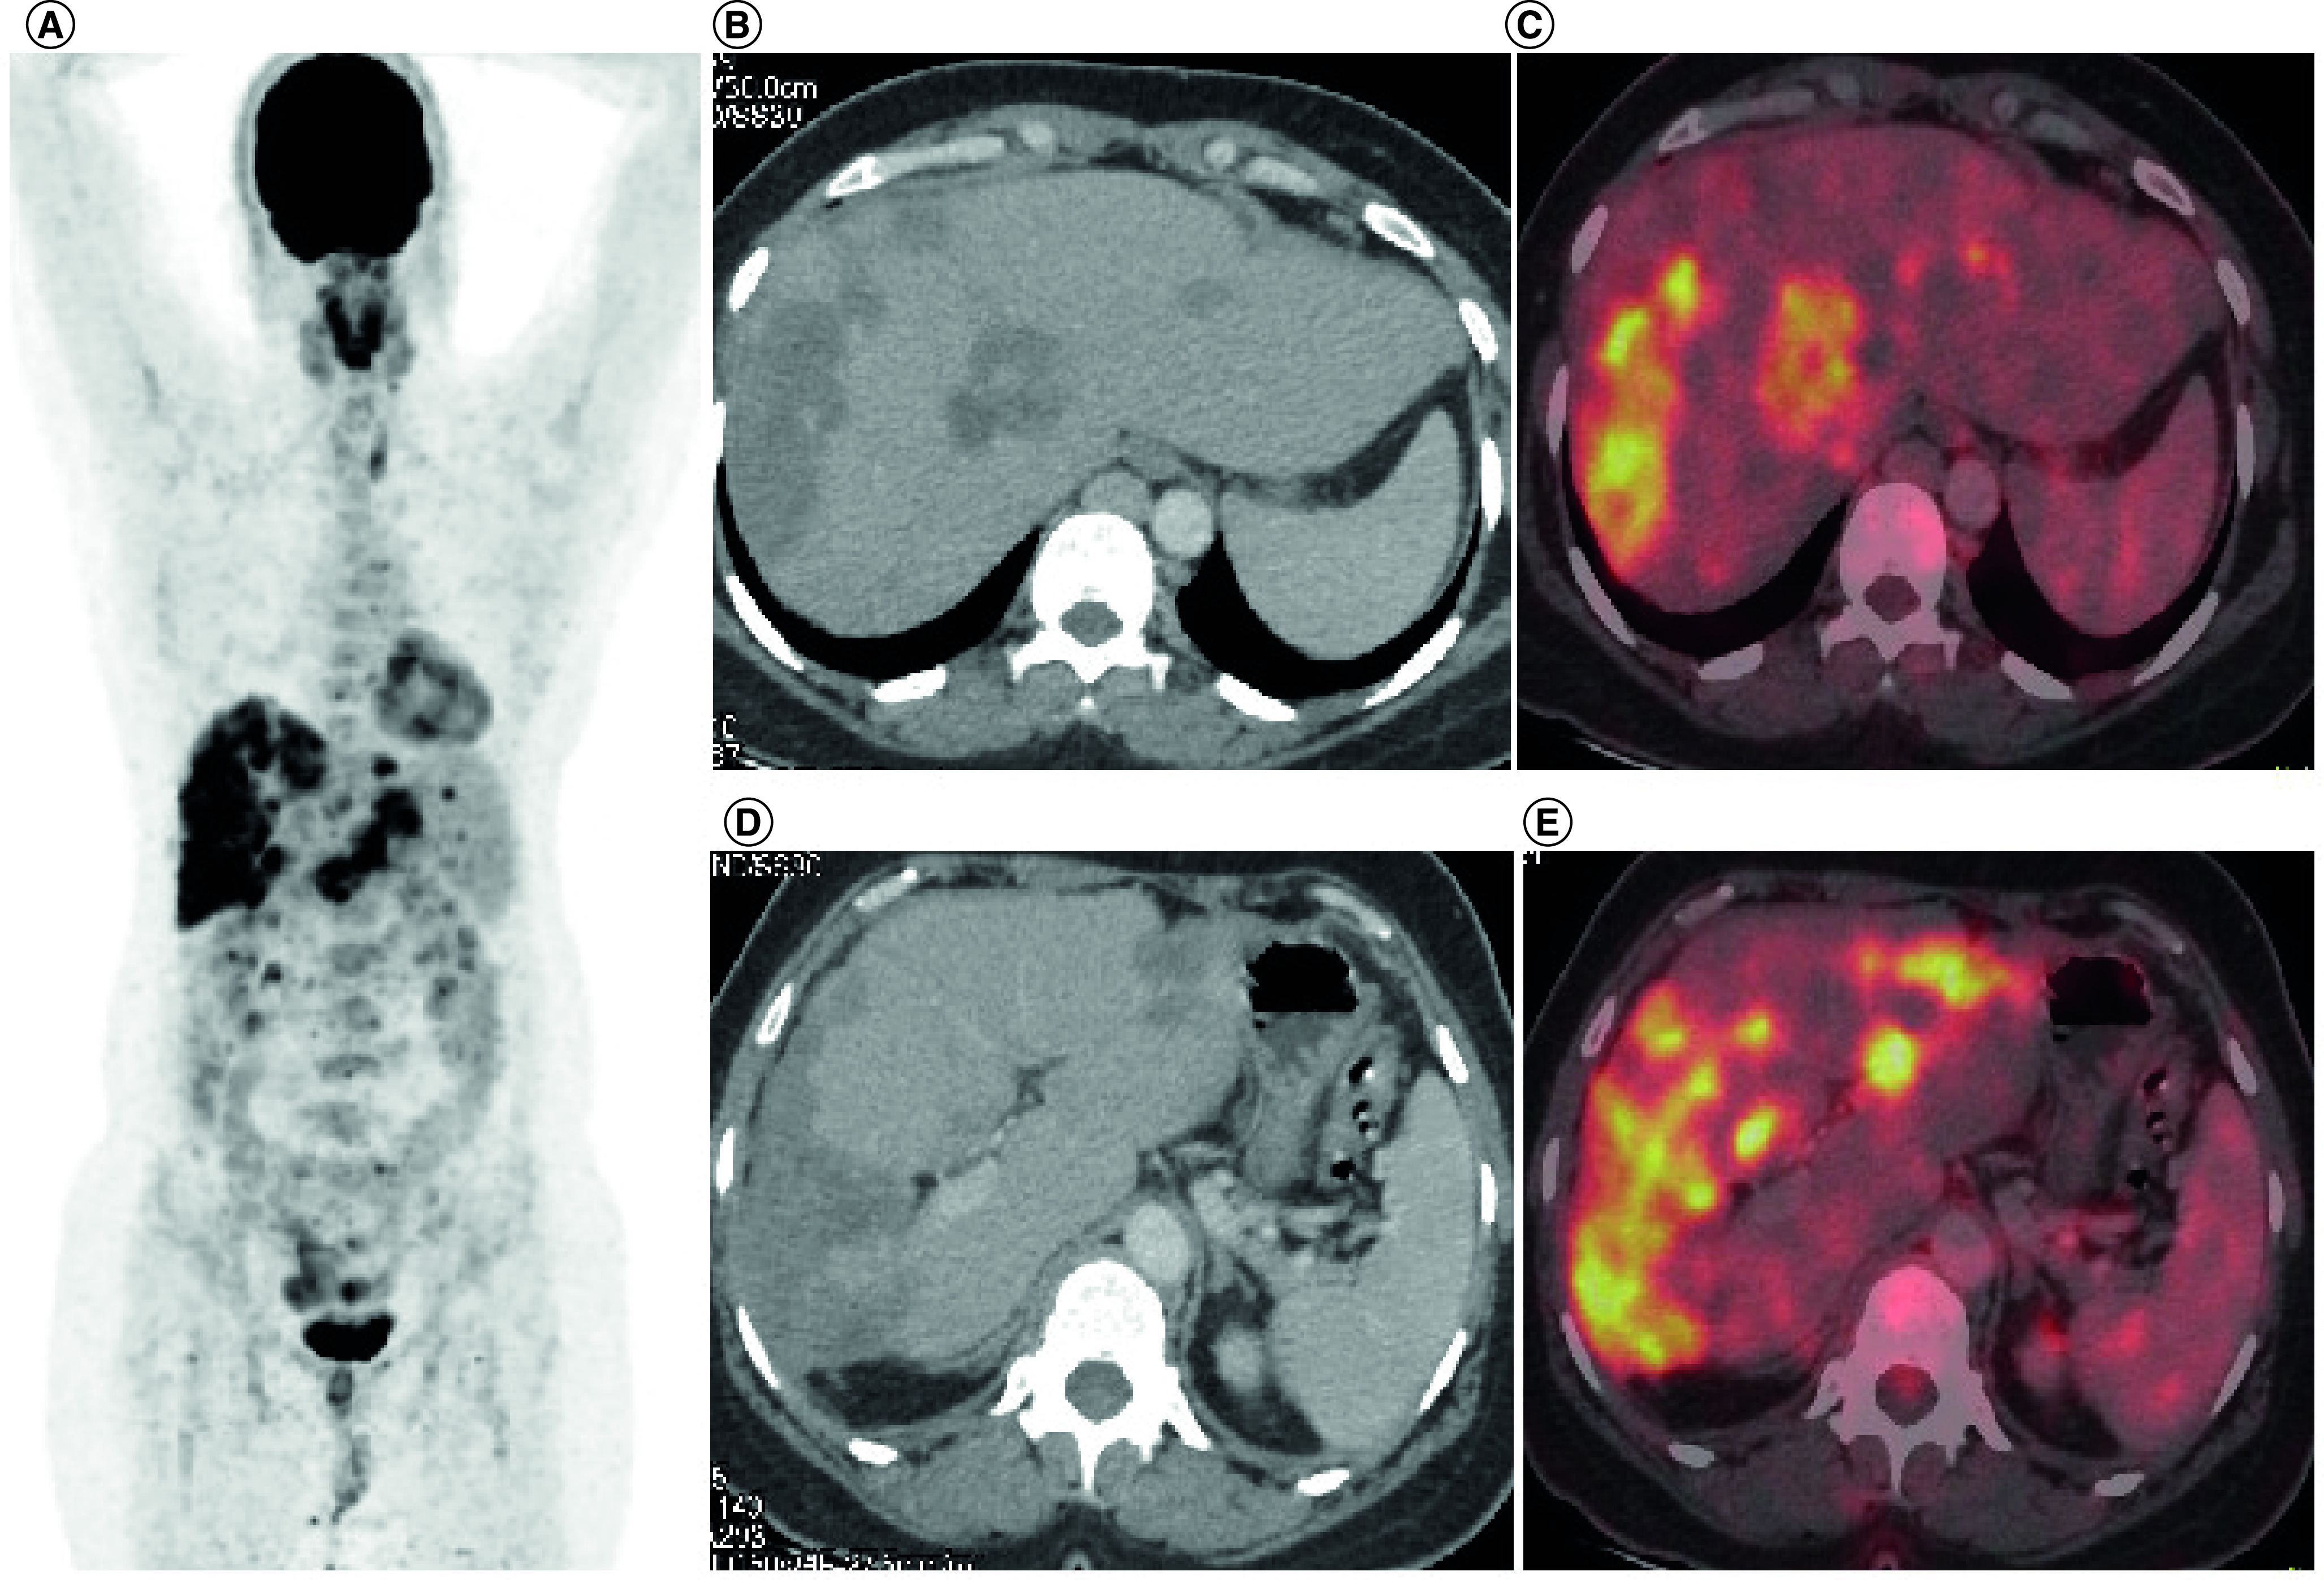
Fluorodeoxyglucose-positron emission tomography images of a 49-year-old female patient of metastatic hepatic epithelioid hemangioendothelioma. This scan represents the radiological findings prior to initiation of pazopanib-based therapy for this patient. (A) MIP image FDG PET-CT showing increased tracer uptake in the hepatic region. (B & D) Axial CT abdomen showing confluent hypodense lesions in both the lobes of liver. (C & E) There is increased tracer uptake noted at the liver lesions in the fused PET-CT images. MIP: Maximum intensity projection.
